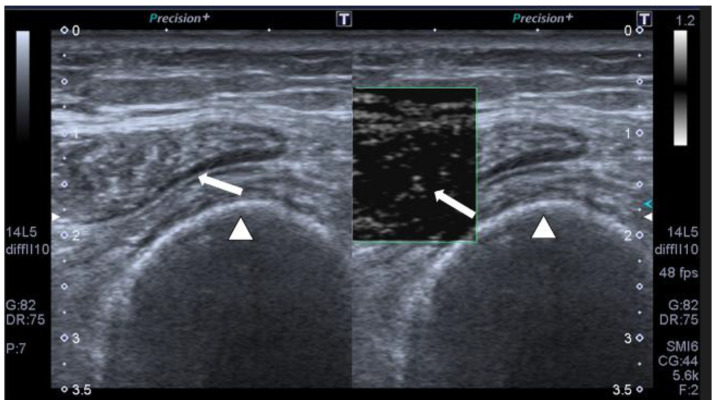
SMI monochromatic mode image shows minimal linear vessels in a 56-year-old patient with multiorgan failure on transverse muscle ultrasound QRF (arrow), femur (arrowhead).
